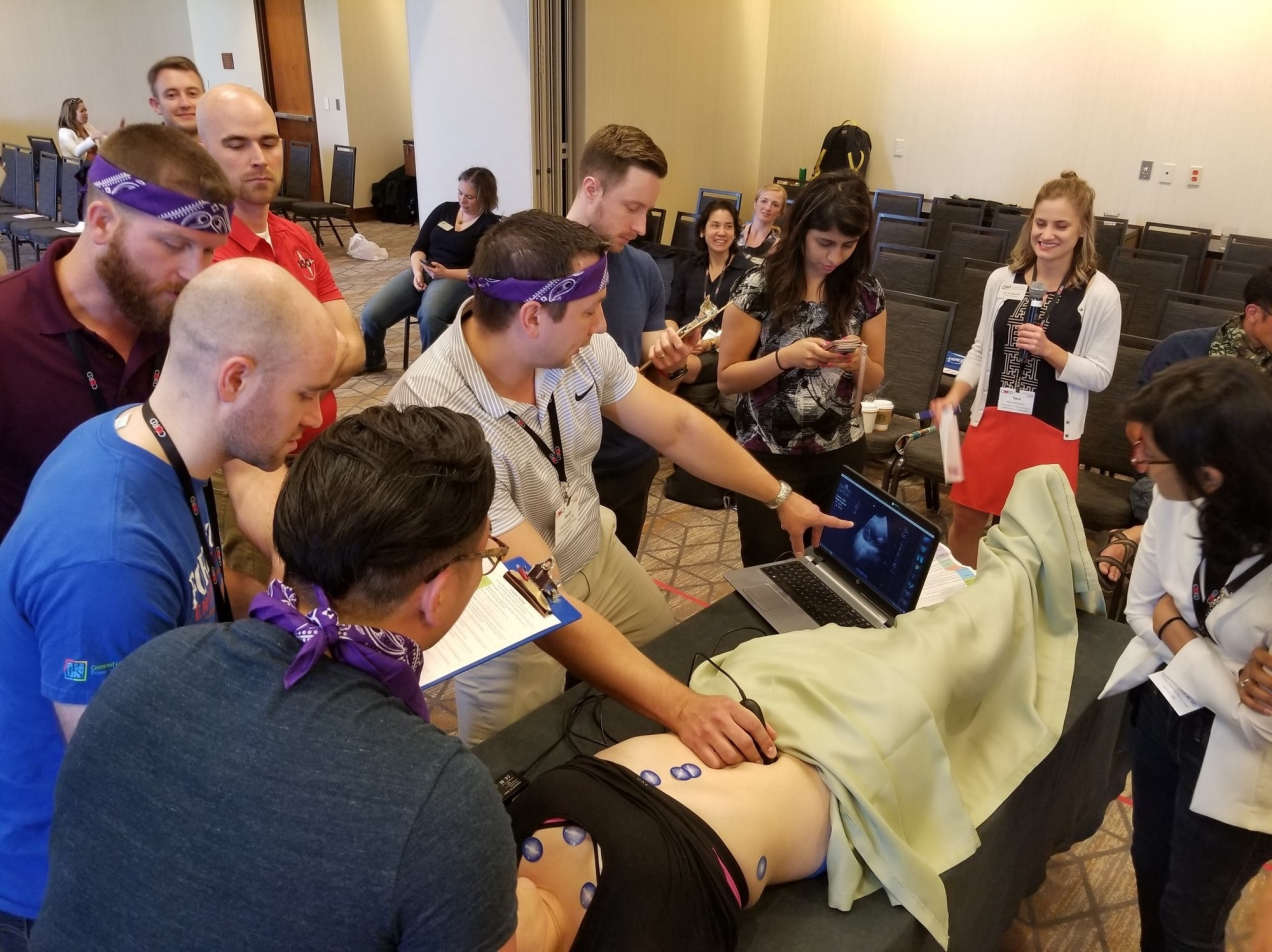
Participants using FUJIFILM SonoSim live scan technology to ultrasound pathologic images on a real patient used specially designed electronic stickers as part of Round 2B. Images of an aorta can be seen on the screen.
